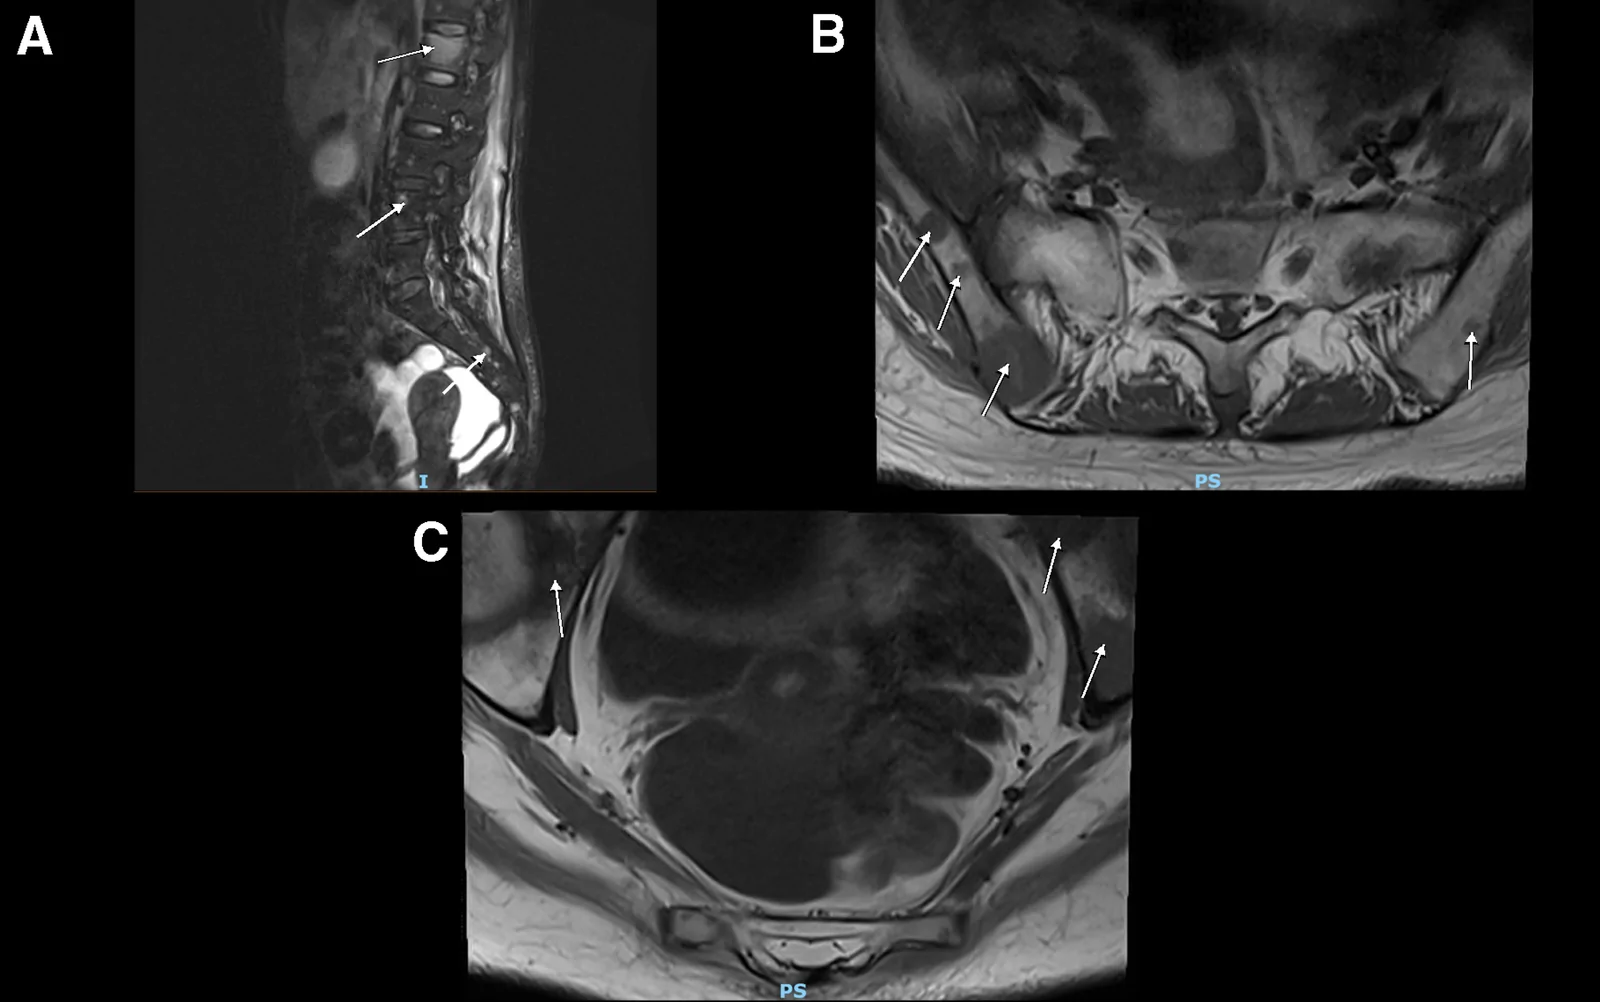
MRI findings before the third radiotherapy course. (A) Sagittal fat-suppressed T2-weighted MRI obtained on April 22, 2024, demonstrates representative abnormal marrow-signal lesions at L1, L4, and S3 (arrows). (B) Axial pelvic MRI demonstrates representative bilateral iliac marrow-signal abnormalities (arrows). (C) Axial pelvic MRI demonstrates representative bilateral acetabular marrow-signal abnormalities (arrows). The arrows identify representative abnormalities and are not intended to indicate the complete extent of osseous involvement. MRI = magnetic resonance imaging.
